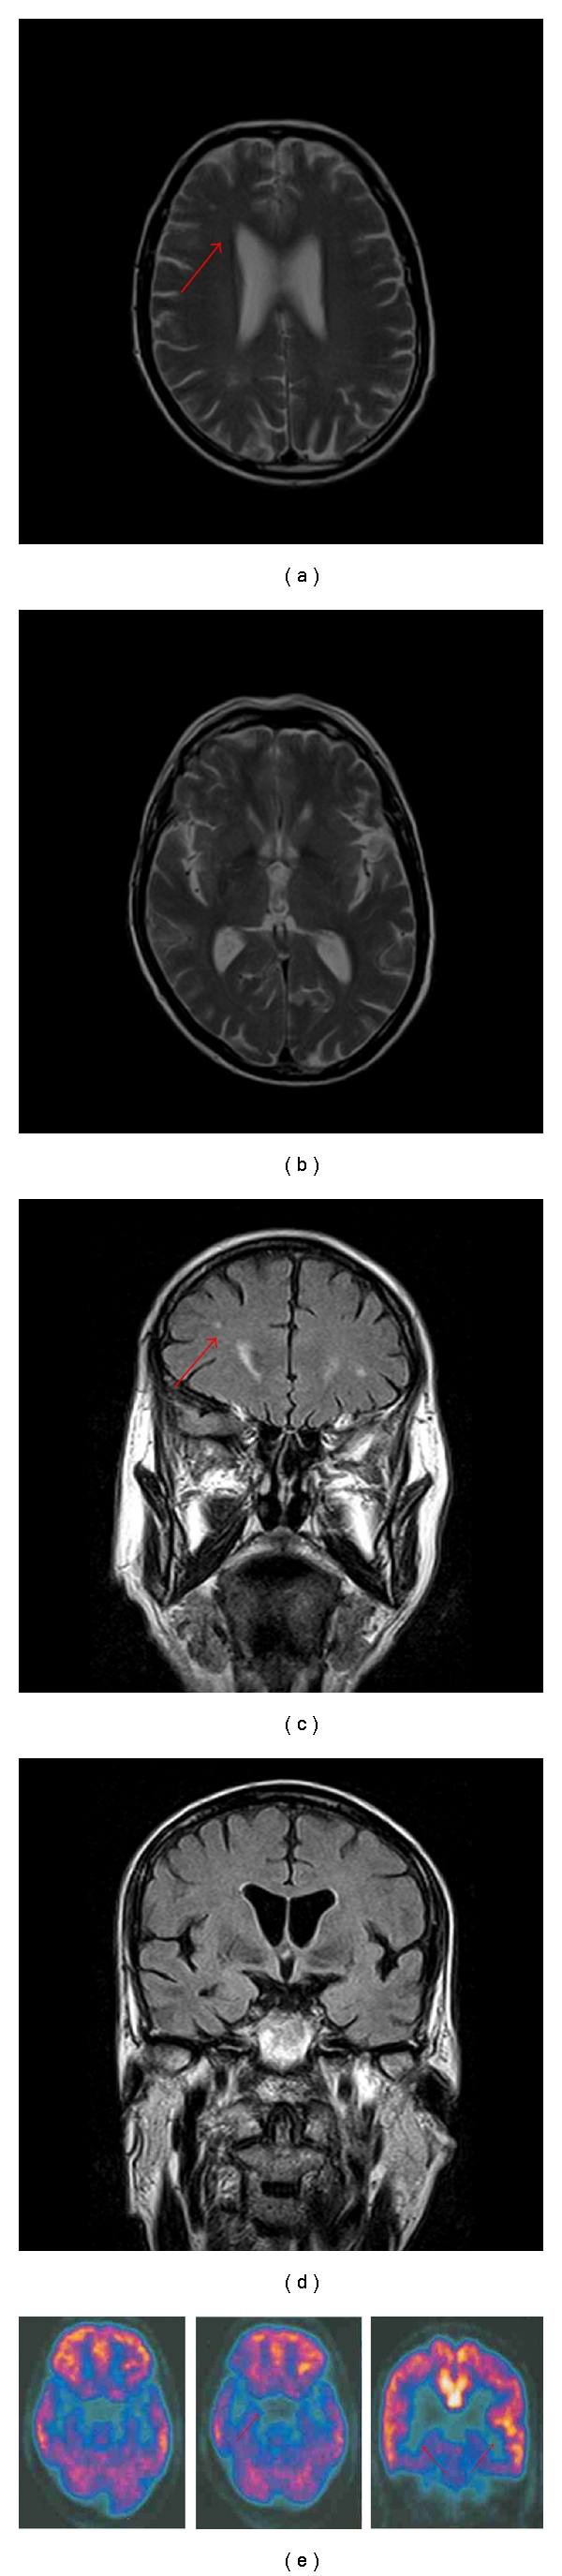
Cerebral MRI axial PD/T2weighted (a, b) and coronal FLAIR (c, d) sequences showing small bilateral aspecific white matter lesions (a, c) involving the frontal white matter (red arrows) and sparing the basal ganglia (b, d). (e) Axial and coronal cerebral FDG PET revealing a metabolic decrease in the brainstem (pons and mesencephalon) and medial temporal cortex (red arrows).
